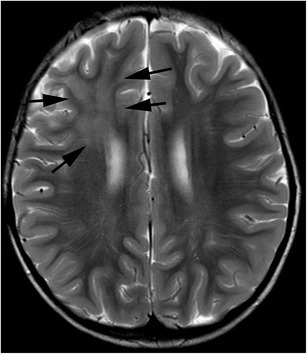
Radiation induced white matter injury. A 5-year-old boy, who received radiation therapy for anaplastic ependymoma, was asymptomatic. A T2-weighted image shows hyperintensity in the right frontal lobe which was irradiated (*arrows*)
